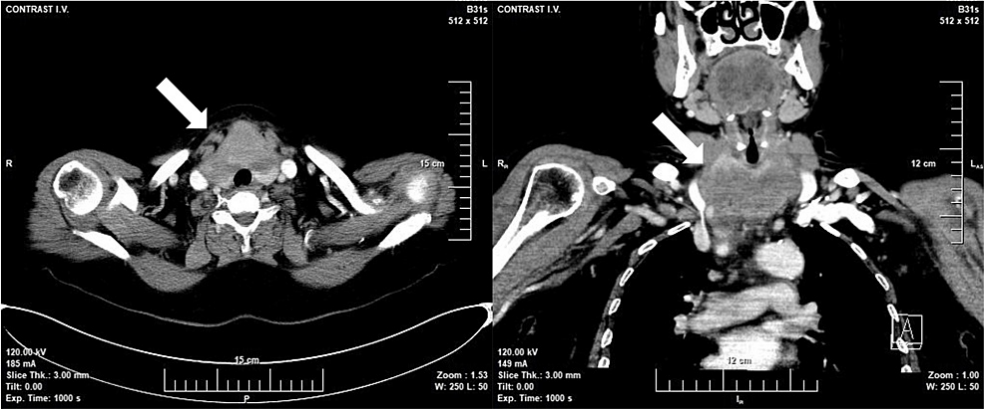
Neck CT at presentation showing axial and coronal sections of the tumor formation (arrow)
